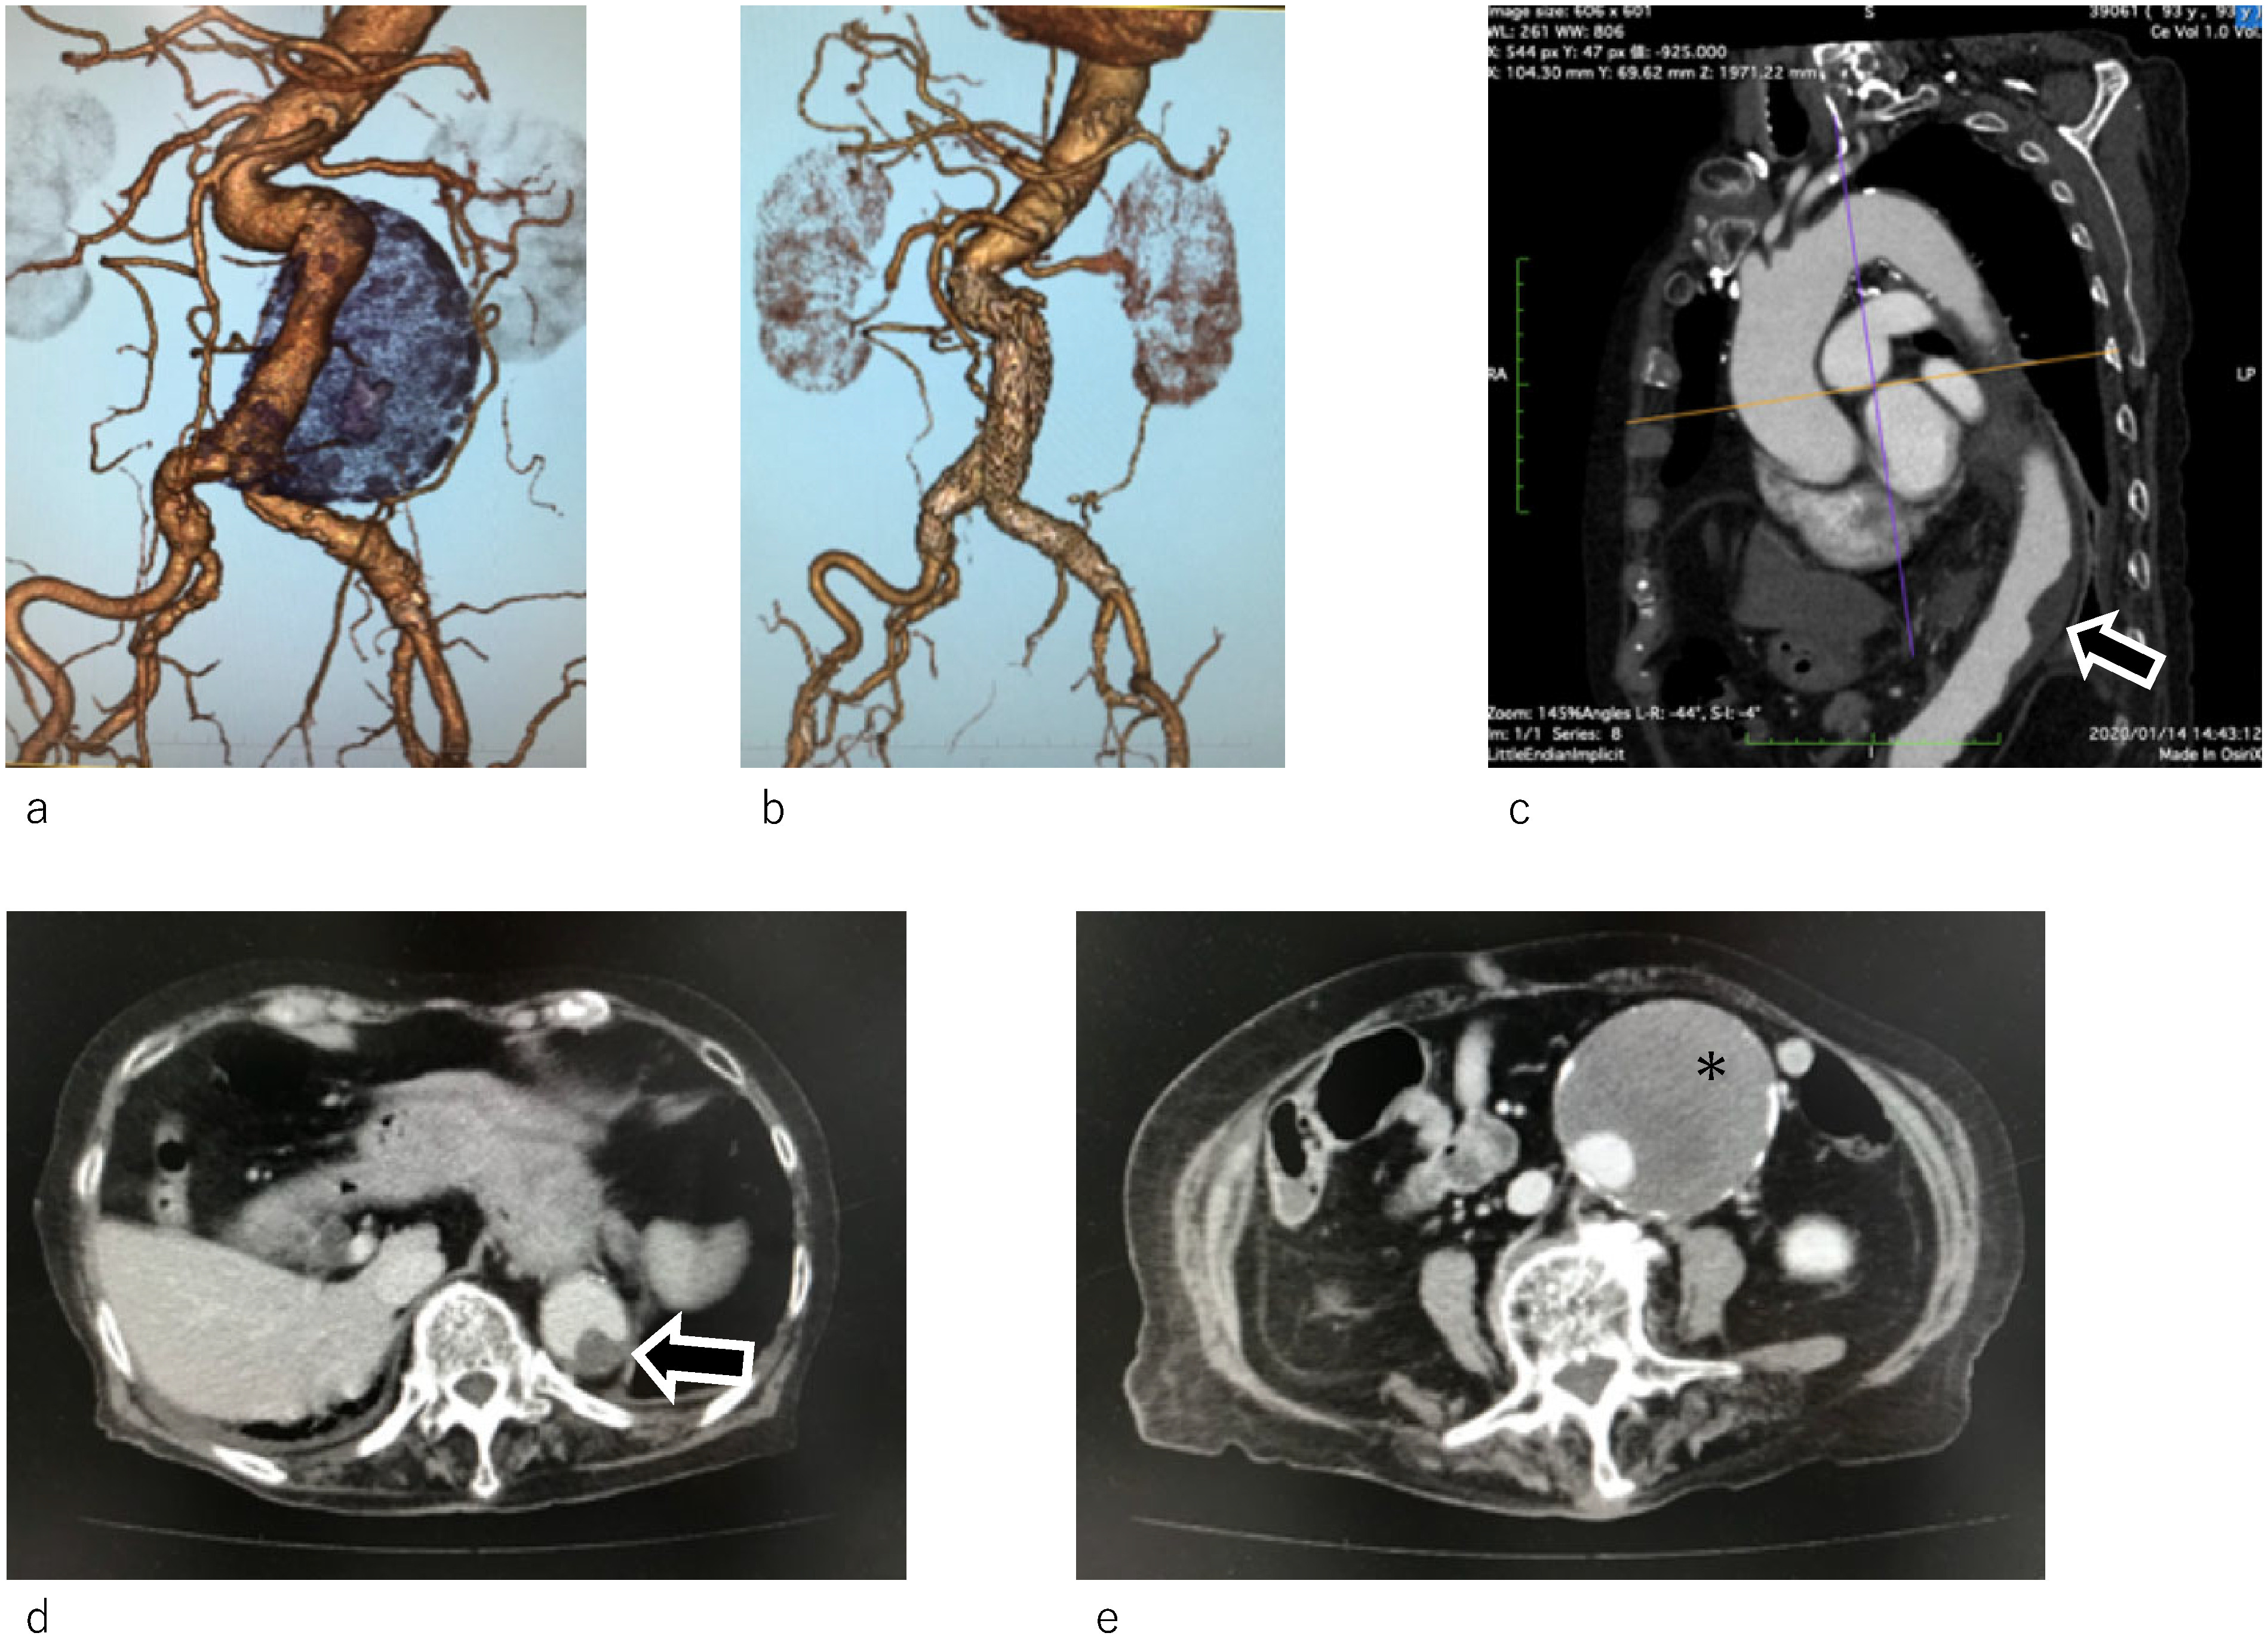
Fig. 1 Pre- and postoperative computed tomography (CT) images: (a, b) Preoperative and postoperative enhanced CT three dimensional images. We successfully placed the stent-grafts as planned. (c–e) Preoperative CT images for analysis of the aorta. We observed a large thrombus in the descending aorta (arrow) and in the aneurysm(*).
